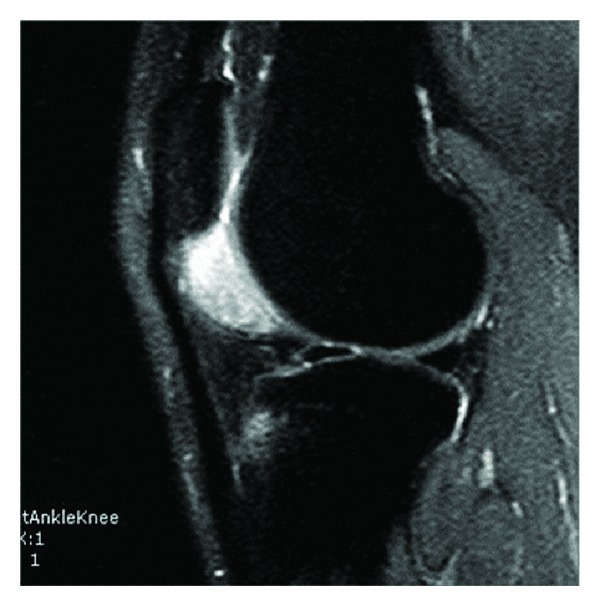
Hyperintense lesion in infrapatellar region on T2-weighted MRI sequences.
